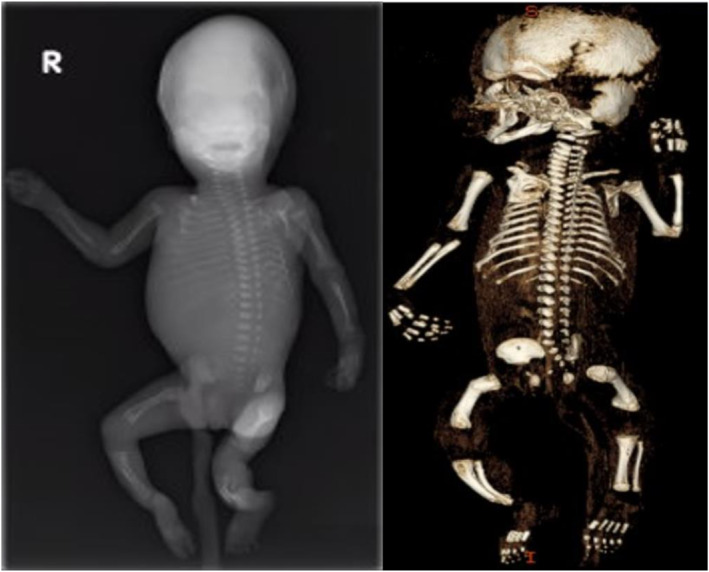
X‐ray and 3D‐CT images of a fetus (H20 + 1) with COL1A2‐related osteogenesis imperfecta after termination. Multiple rib fractures, short femora with fractures and bowed tibia can be seen.
